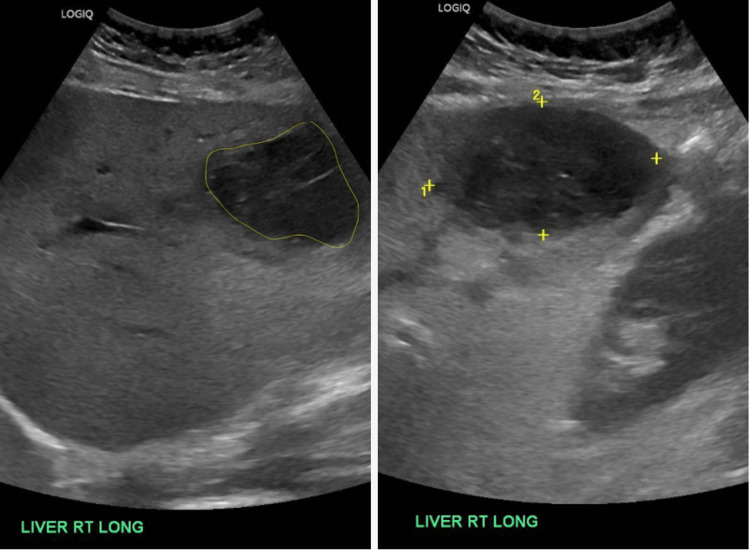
Sonogram demonstrating heterogeneous right hepatic mass (circles on left, and hatchmarks on right) and an additional smaller hypoechoic lesion, suspicious for underlying malignancy.
